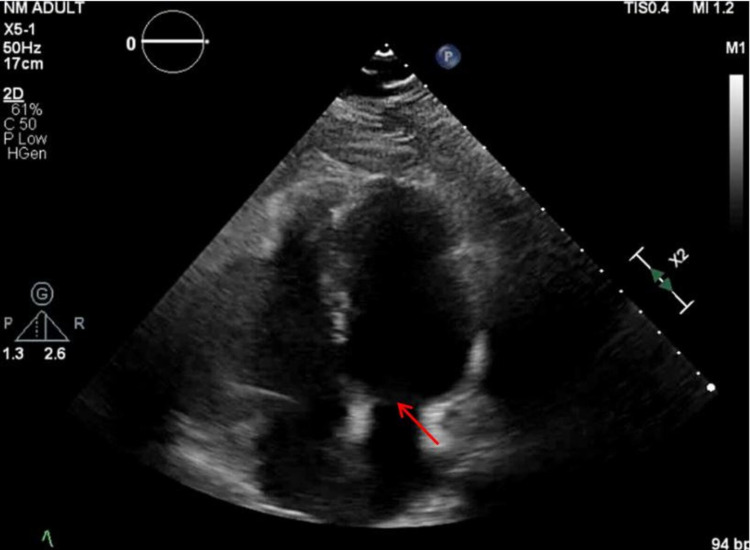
Two-dimensional TTE with Doppler in four-chamber view showing normal structure of the heart. There is no evidence of left ventricular hypertrophy. The left ventricular systolic function is normal with an estimated ejection fraction of 60%-65%. The trivial to mild mitral valve prolapse (arrow) remains stable compared to prior study. TTE - transthoracic echocardiogram
